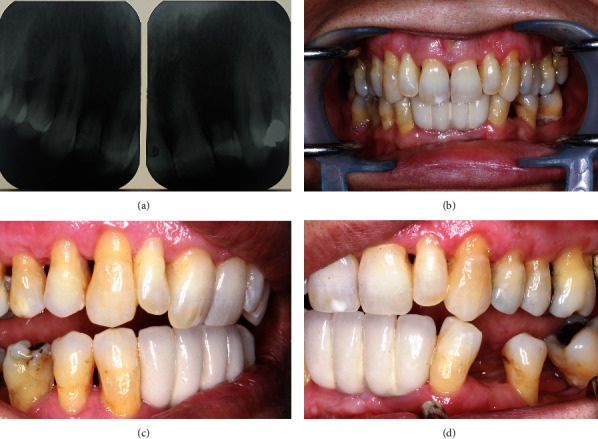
One-year follow-up. (a) X-ray radiography. (b) Retracted view. (c) Lateral movement in right side. (d) lateral movement in left side.
